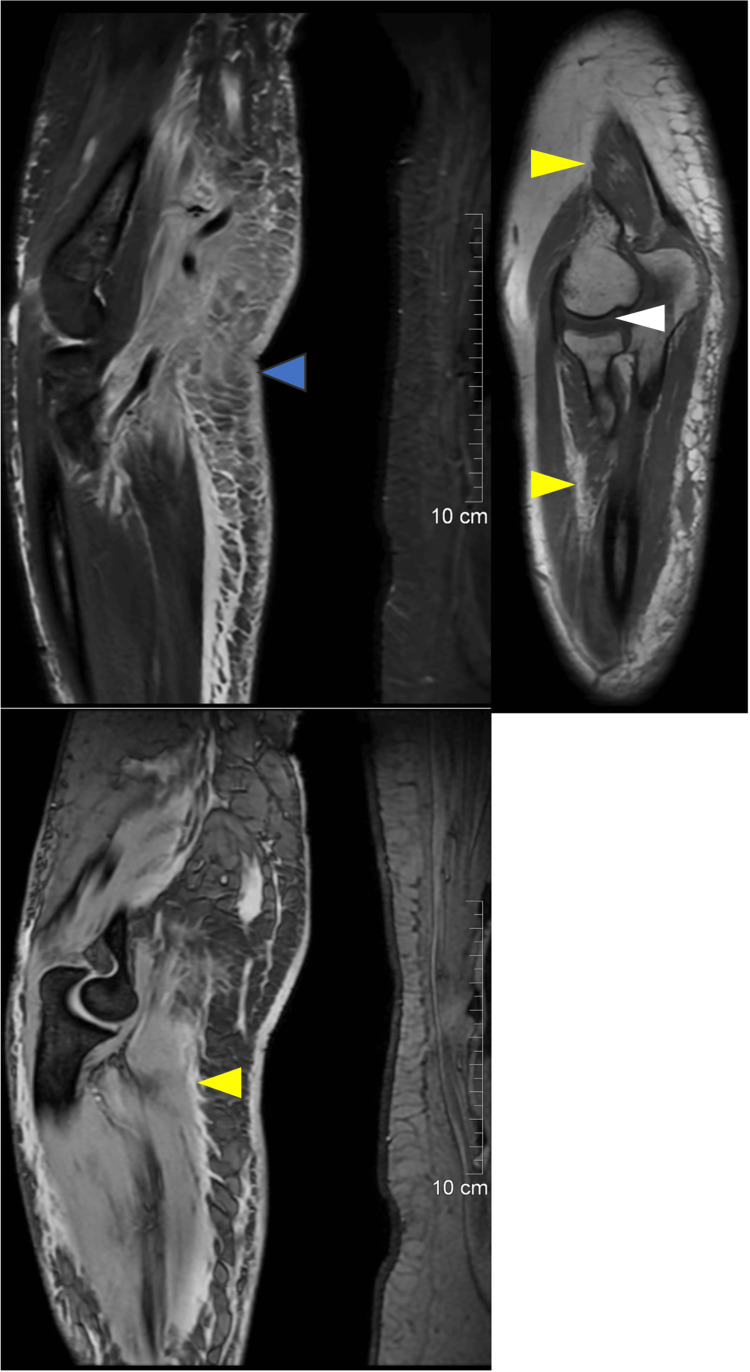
MRI elbow There is diffuse subcutaneous oedema and post-contrast enhancement with associated diffuse, almost circumferential, skin thickening (blue arrow). There is intramuscular edema and postcontrast enhancement of the anterior compartment muscle, mainly involving the distal portion of the brachialis and biceps muscles (yellow arrows). In addition, there is intramedullary bone marrow edema of the distal metadiaphysis of the humerus and proximal metadiaphysis of both the ulna and radius with post-contrast enhancement (white arrow).
